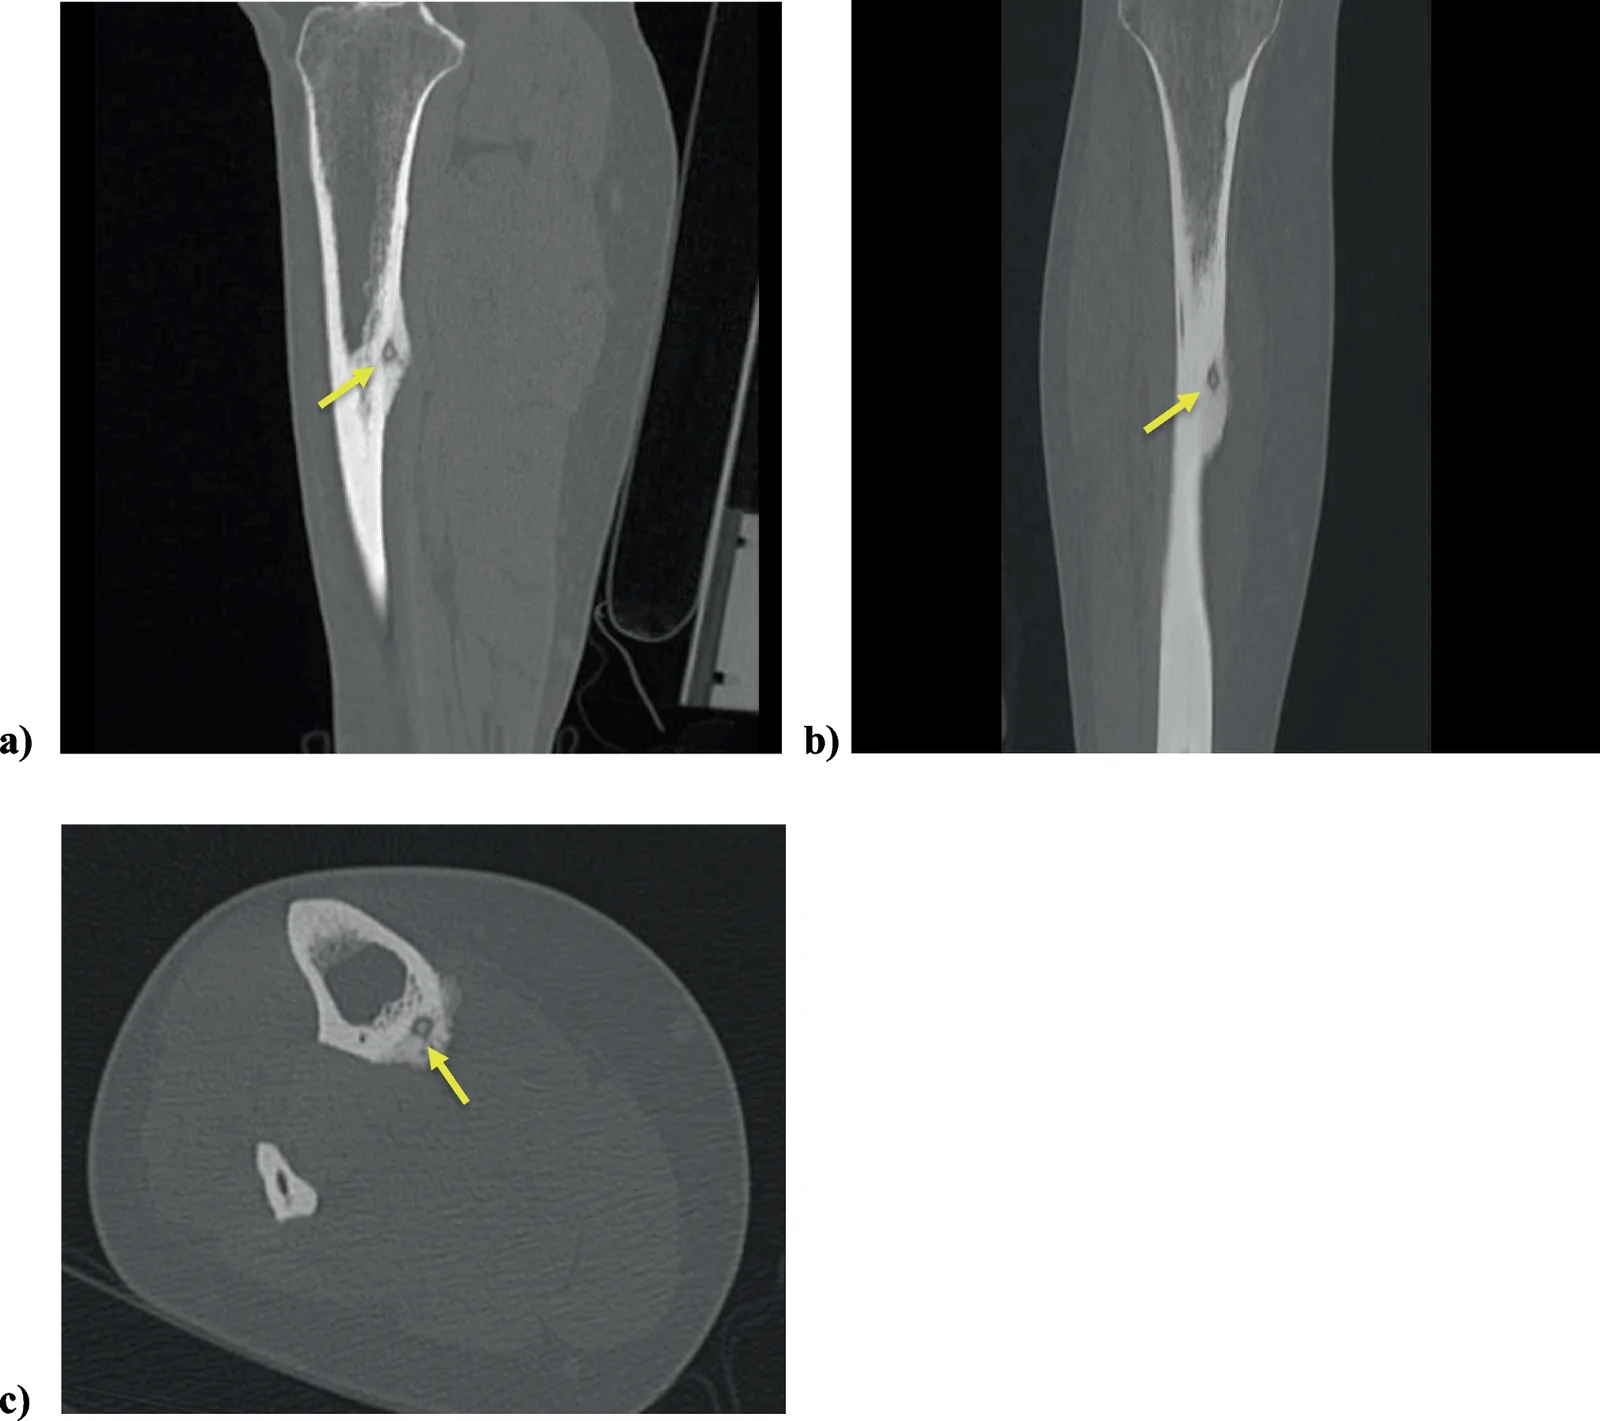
Multiple CT images of the right tibia and fibula demonstrating a subperiosteal nidus along the posterior medial mid tibial diaphysis with central calcifications and associated cortical thickening and periosteal bone formation in a sagittal, b coronal, and c axial views
